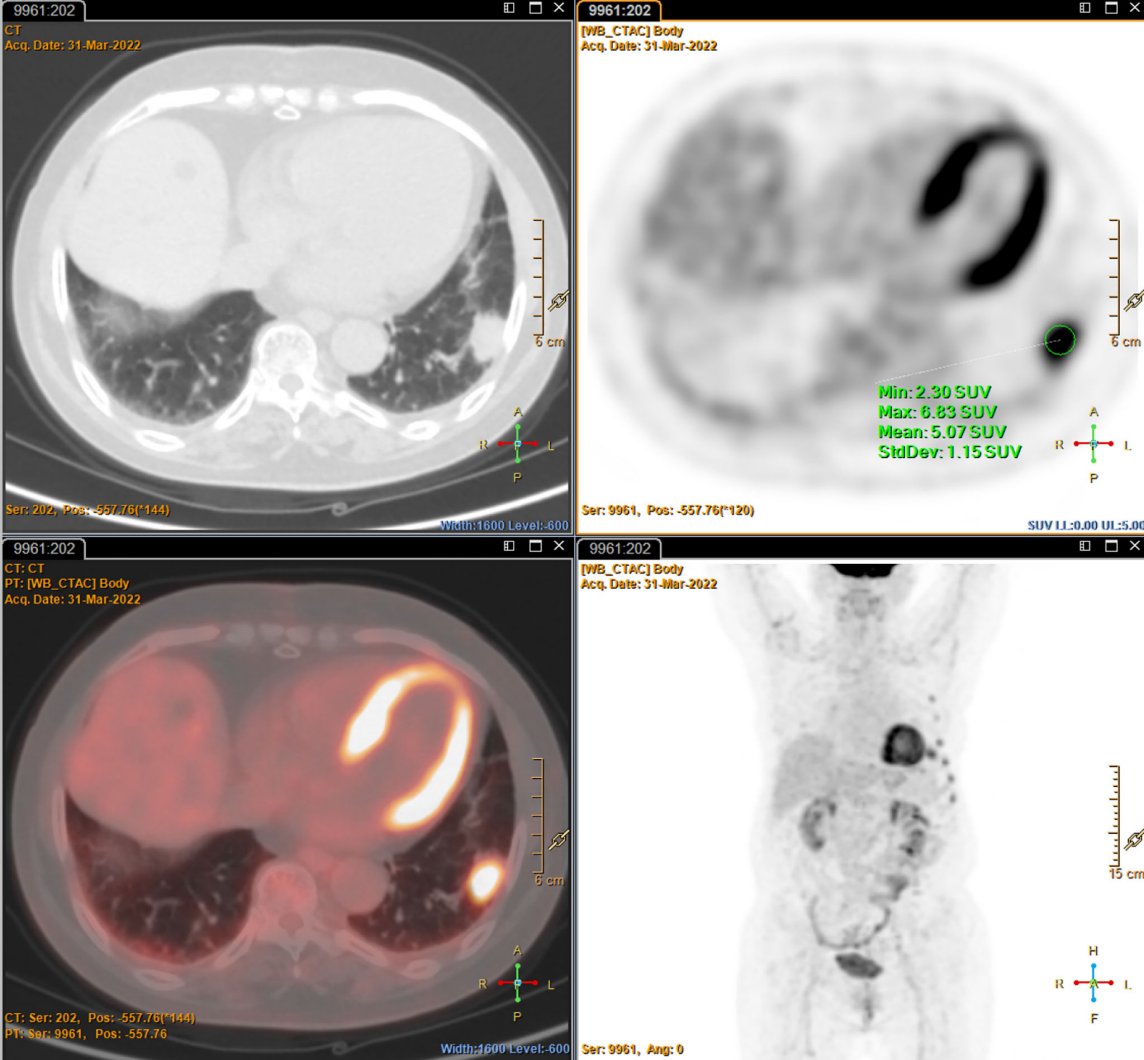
The Positron emission tomography-CT imaging characteristics shows a soft tissue density with hypermetabolism in the left lower lobe, but no abnormal metabolism in other organs and tissues, and no osteolytic lesions.
